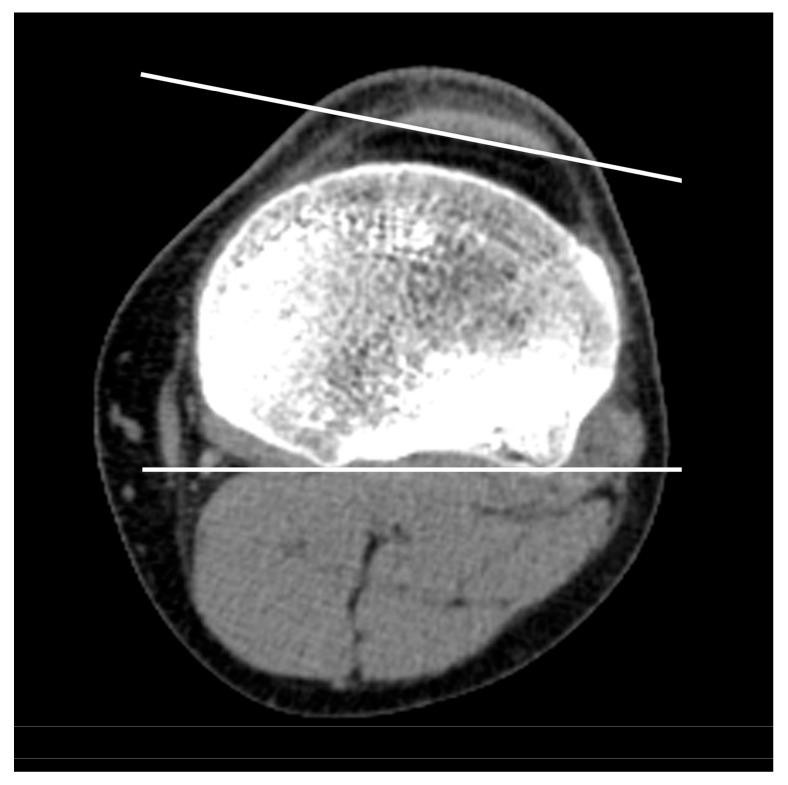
Tendon yaw (TY) angle measured on the first ascending CT image where the fibula was no longer visible (“headless cut”).
